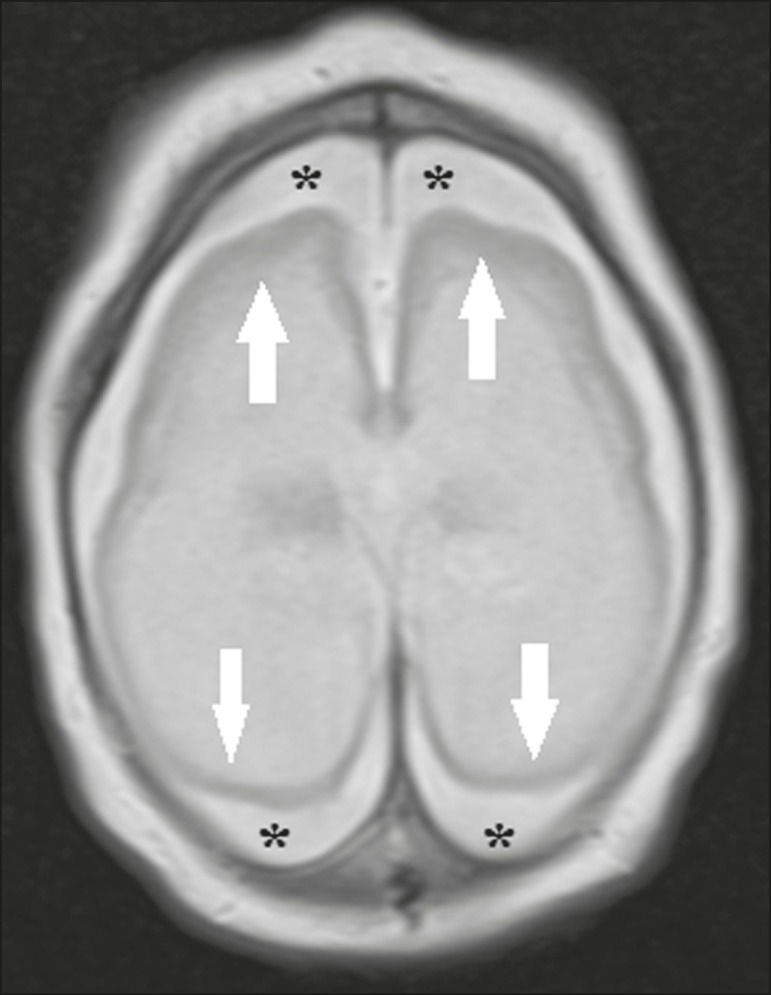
A 2-month-old patient. Axial T2-weighted MRI slice showing marked
